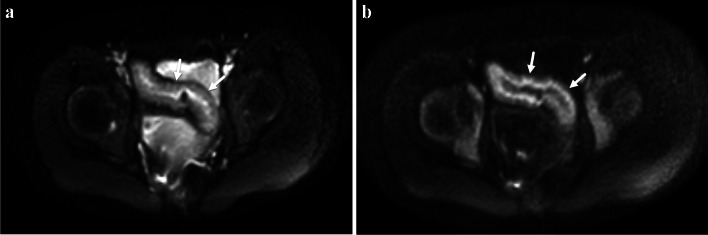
A 14-year-old male with ileal Crohn’s disease. a Axial low *b*-value (*b* = 0 s/mm^2^) diffusion-weighted MR image shows wall thickening of the terminal ileum (*arrows*). b Axial high *b*-value (*b* = 800 s/mm^2^) diffusion-weighted MR image shows restricted diffusion of water within the terminal ileum suggestive of active inflammation (*arrows*). This portion of the bowel appeared hypointense on the corresponding apparent diffusion coefficient (ADC) image
